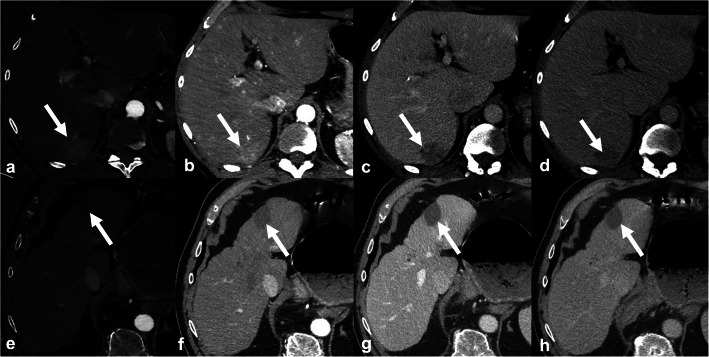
Dual-energy computed tomography (DECT). a-d 58-year-old man with 39-mm hepatocellular carcinoma (arrows) imaged with DECT. Arterial phase hyperenhancement is better visualized on iodine map (a) than on the standard late hepatic arterial phase (b). Lesion also shows washout on portal venous (c) and delayed phase (d). e-h 79-year-old man with hepatocellular carcinoma treated with microwave ablation (arrows) and imaged with DECT. Iodine map (e), standard late hepatic arterial phase (f), portal venous (g), and delayed phase (h) demonstrate no residual enhancement consistent with complete tumor treatment
